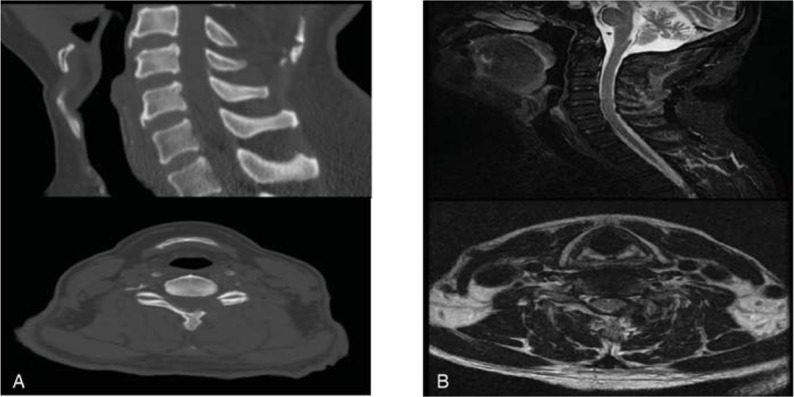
Postoperative images of CT (A) and MRI (B): postoperative images showed abnormal ligamentum flavum were removed, indicating a significant decompression.
